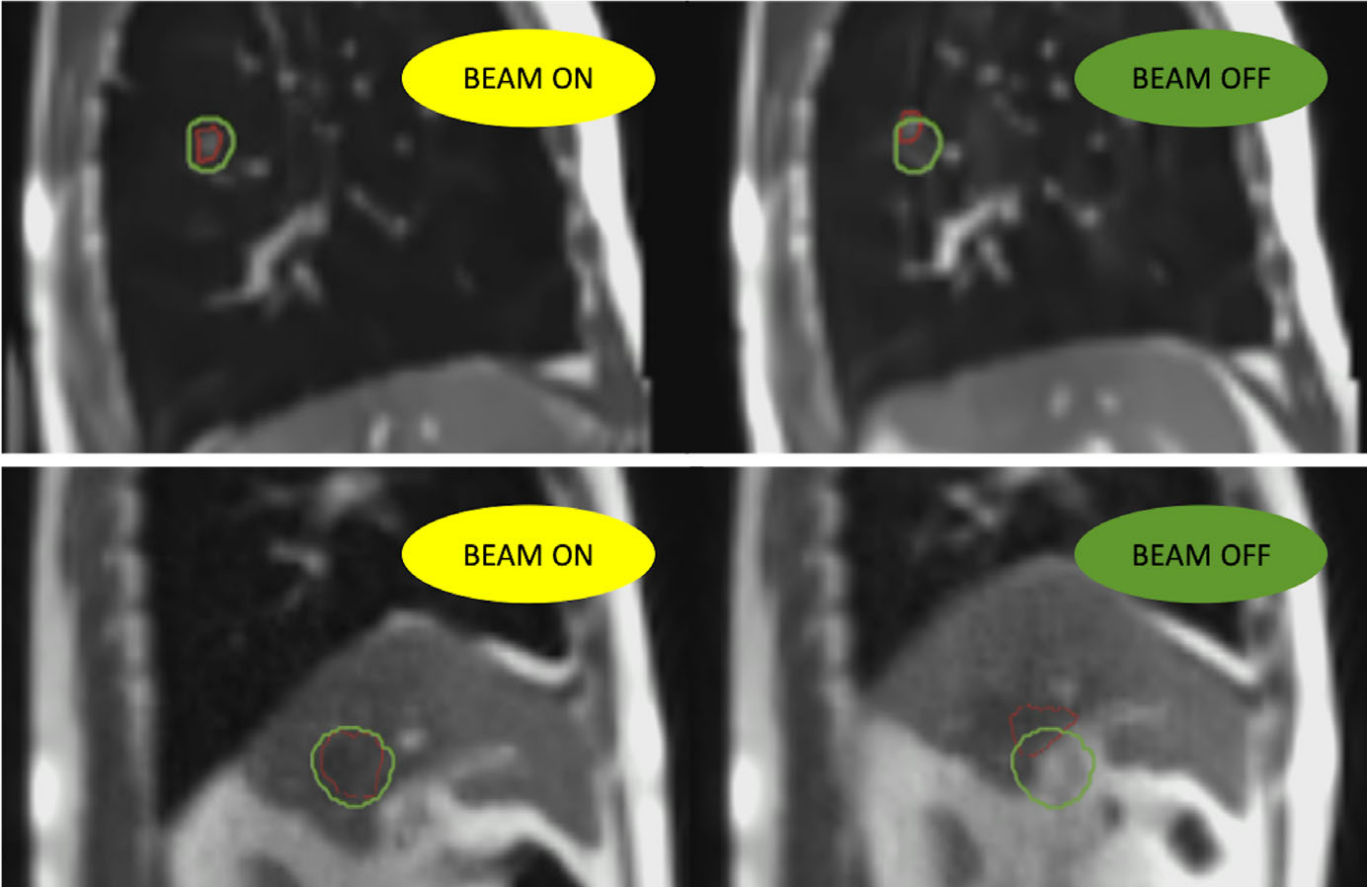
Real‐time sagittal MRI acquisition during treatment delivery (top images is a lung case, bottom images is a liver case): target (red) is irradiated (BEAM ON) only when it is located within the boundary (green). If a defined percentage of the target is outside of the boundary, delivery is automatically interrupted (BEAM OFF).
